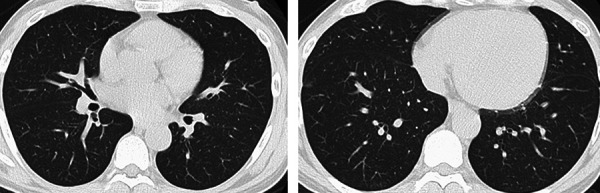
Alveolar bleeding gradually disappeared in the chest computed tomography on day 35.
